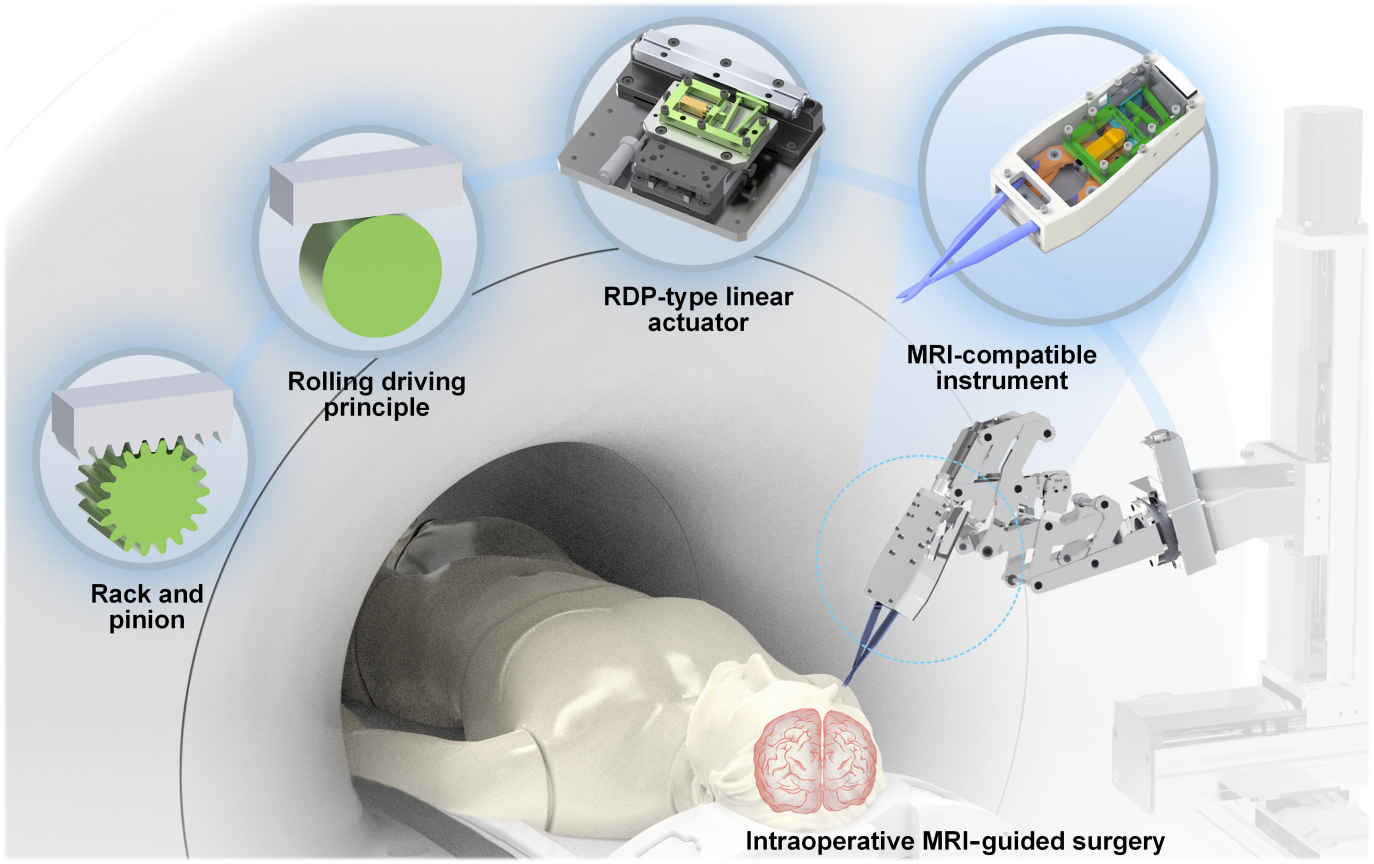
The proposed RDP-type stick-slip actuator and its application in MRI-compatible instruments with great potential for intraoperative MRI-guided surgery.
